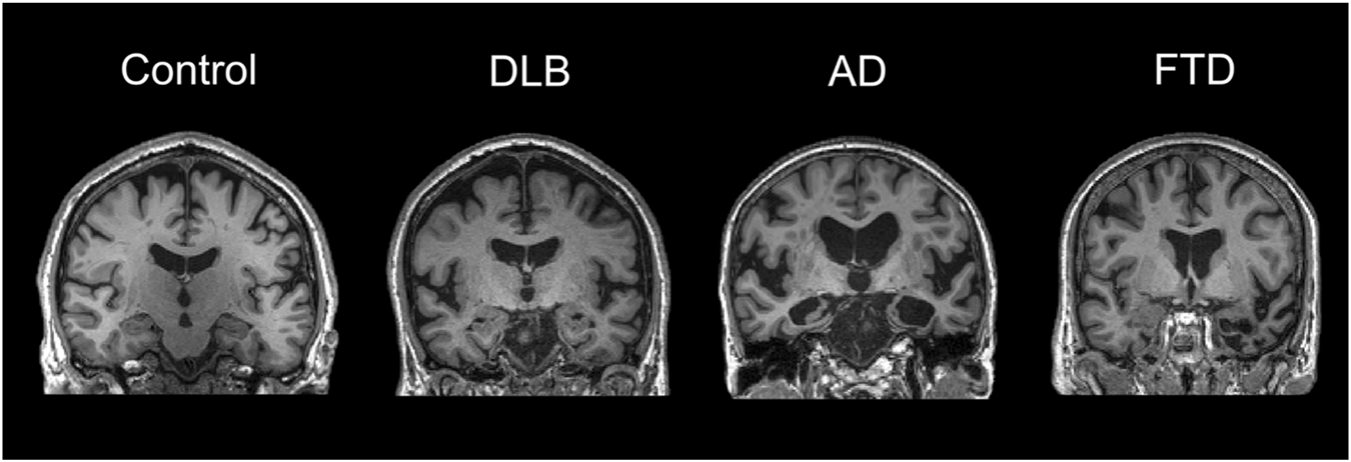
Representative MRI scans in different types of dementia. The figure shows representative MRI scans from a non-demented control and from patients with Dementia with Lewy Bodies (DLB), Alzheimer’s disease (AD) and frontotemporal lobe degeneration (FTLD). It highlights the characteristic patterns of atrophy with relative preservation of the hippocampus in DLB, severe hippocampal atrophy in AD and temporal pole atrophy in FTLD. These scans are from the Neuroimaging of Inflammation in Memory and Other disorders (NIMROD) study cohort. Images are courtesy of Dr Elijah Mak, University of Cambridge, UK.
